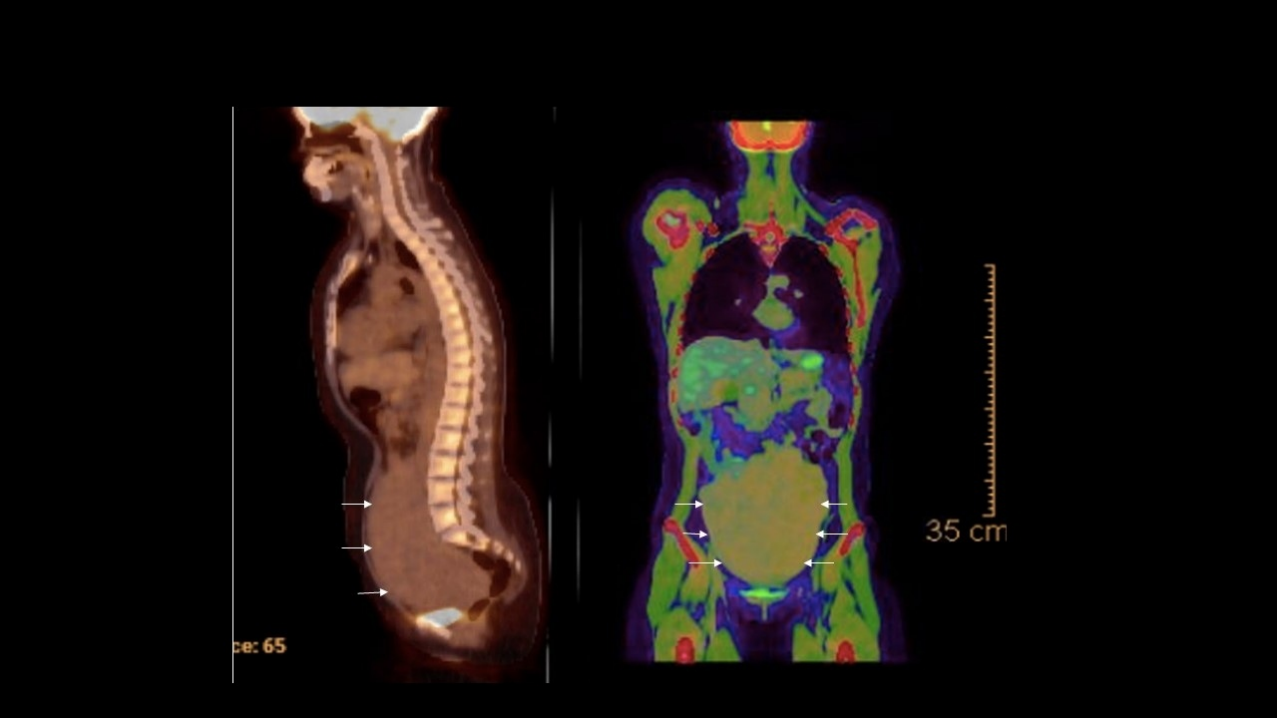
PET CT of patient shows a large mass in the pelvis (white arrows) PET: positron emission tomography; CT: computed tomography
